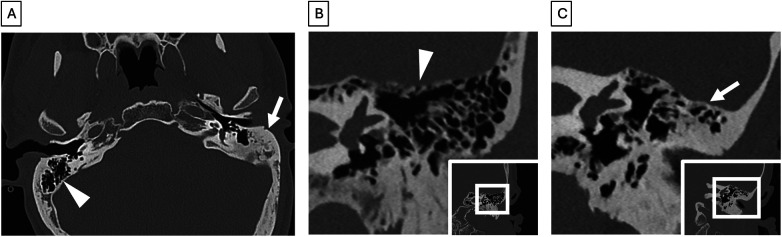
Mastoid anatomy (all images represent different patients). Axial CT shows highly pneumatized mastoid air cells on the right (arrowhead) and sclerotic air cells on the left (arrow)​. (A) Photon-counting coronal CT shows normal tegmen mastoideum (arrowhead) height. (B) Photon-counting coronal CT shows reduced tegmen mastoideum (arrow) height.
